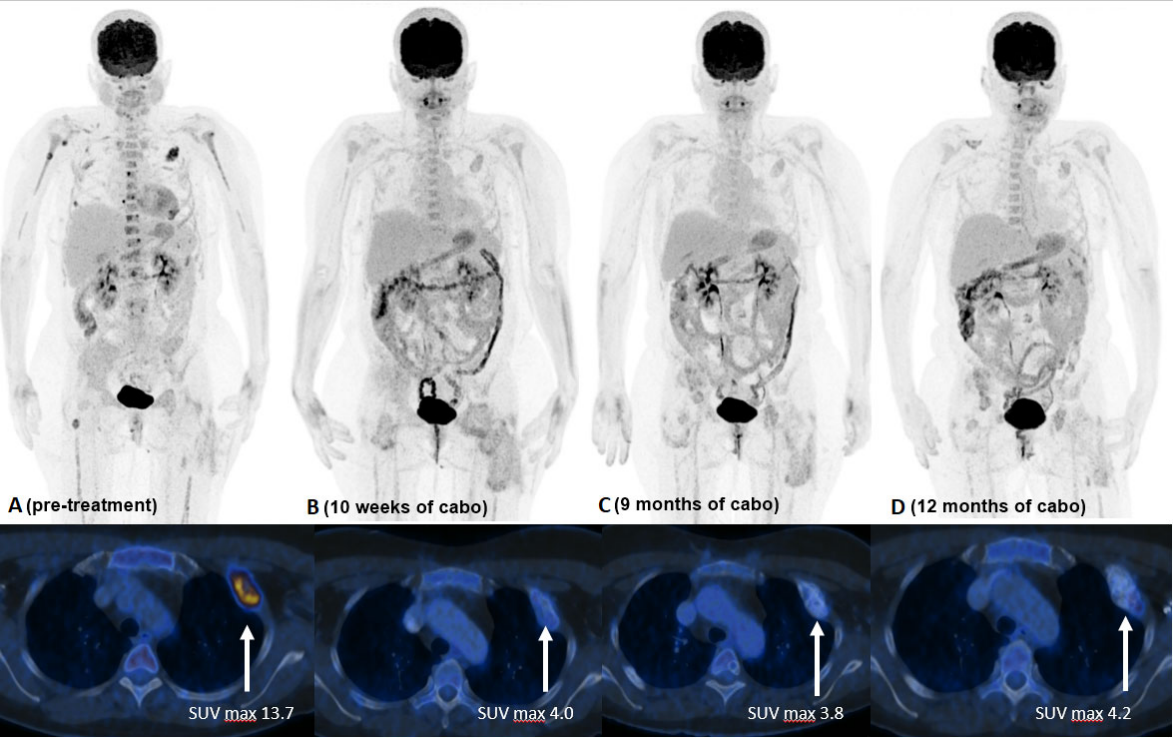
FDG PET/CT [(A) 7/21] with multiple FDG avid bone metastases (ribs, spine, femurs, humerus, skullcap). SUV max, in the large left sided rib metastasis 13.7. FDG PET/CT [(B) 10/21] 10 weeks after start of TKI treatment with reduction of uptake in all bone metastases (rib lesion reduction to SUV max. 4.0). No new FDG avid lesions visible. FDG PET/CT [(C) 4/22] 9 months and [(D) 7/22] 12 months after TKI start with ongoing good response.
